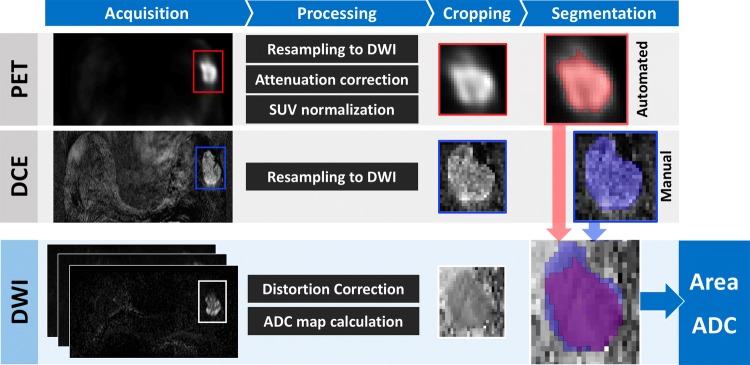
All images were resampled to diffusion-weighted imaging (DWI) resolution. PET images were attenuation-corrected and SUV normalized. Apparent diffusion coefficient (ADC) maps were calculated from distortion-corrected diffusion images. Tumor segmentation was performed by semi-automated Gaussian mixture modelling (GMM) segmentation on cropped PET images, and manually on DCE images. Resulting regions-of-interest (ROIs) were transferred to the ADC maps for derivation of tumor area and mean ADC
